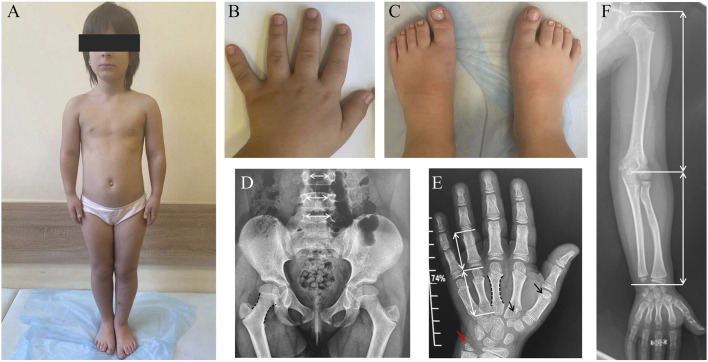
Clinical features of the patient with *PRKG2*-associated acromesomelic dysplasia. (A) General appearance showing disproportionate short stature. (B) Hands with mild brachydactyly and broad, shortened fingers. (C) Feet demonstrating brachydactyly, large and broad great toes and a sandal gap. (D) Antero-posterior radiograph of the pelvis and lumbar spine: in general, the proportions of the spine, pelvic and femoral bones are not disturbed; femoral necks appear slightly elongated, slender and bi-concave (black dashed lines); the interpedicular distances from L3 to L5 progressively increase (normal pattern). (E) Postero-anterior radiograph of the hand: shortening of the metacarpals relative to the phalanges (white arrows); slender, bi-concave contour of the II-IV metacarpals (black dashed lines); incomplete pseudo-epiphyses of the first and second metacarpals (black arrows); elongated styloid process of the ulna (red arrow). (F) Mild mesomelic disproportion in the lengths of the humerus and the forearm (white arrows).
